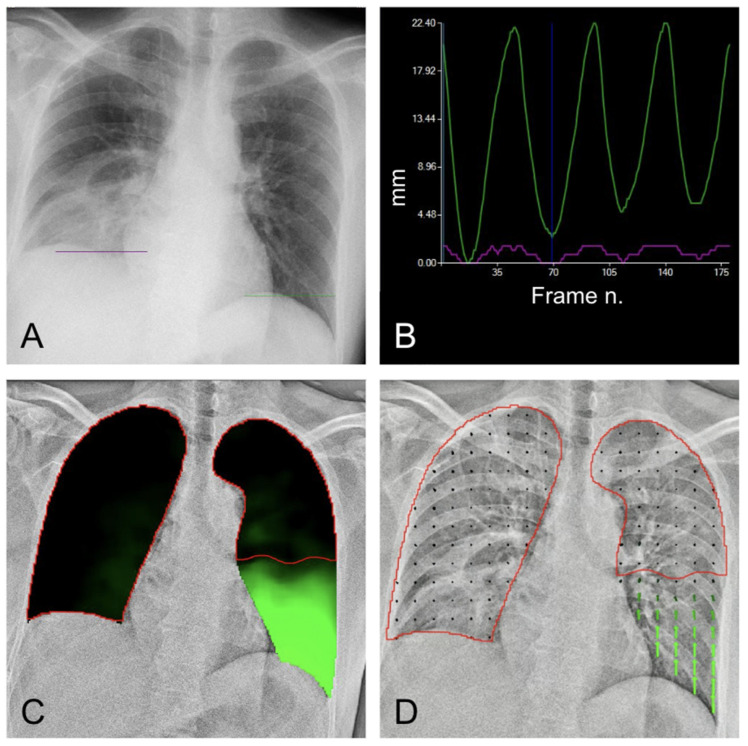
Lung motion analysis. (A) Morphological evaluation of radiographic examination shows elevated right hemi diaphragm and fibrotic bands in the right lower lung field. (B) DM mode shows reduced motility of the right diaphragm. Purple line = right diaphragm; green line = left diaphragm. (C) LM analysis shows poor ventilation of the right lung compared with the left side. (D) Vector maps highlight the lack of mobility in the lower right base (no detectable arrows = no movement).
